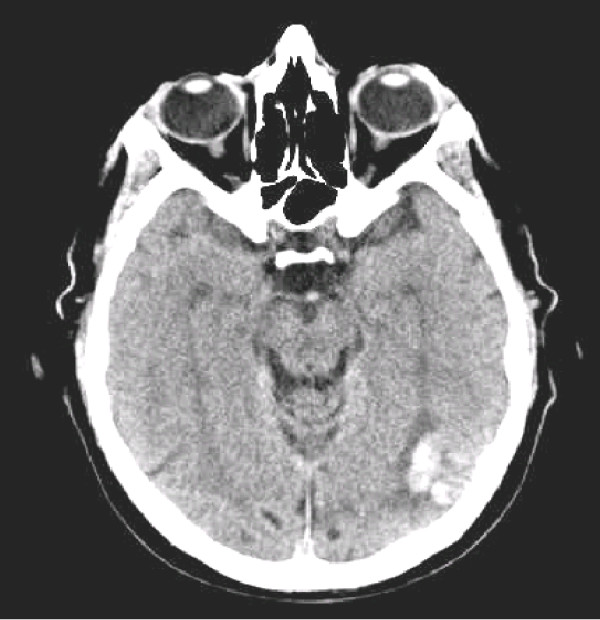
Cerebral computed tomography scan showing a small left cortical temporo-occipital hemorrhage, with mild mass effect and hypodense halo.
